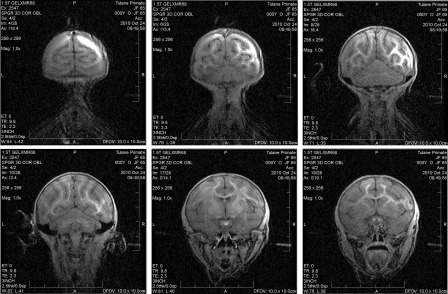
Nuclear magnetic resonance imaging of central nervous system myelin. Coronal images of JF65 at approximately 100 days after direct intracranial mesenchymal stem cell administration to the right brain hemisphere. Images clearly show more pronounced myelination on the injected versus contralateral hemisphere within the occipital white matter, cortical region of the brain, corpus callosum, caudate nucleus, and putamen.
